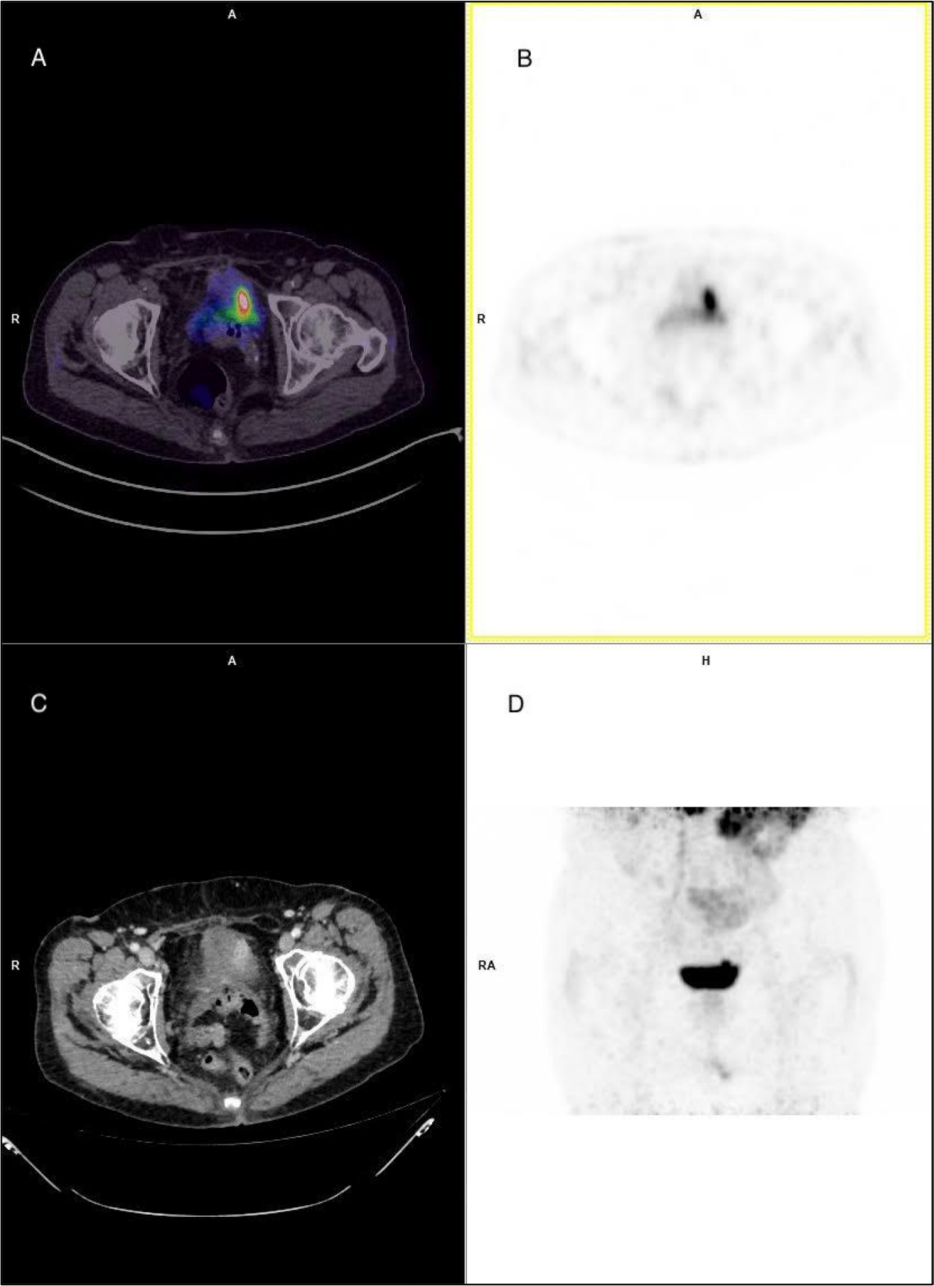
78-year-old patient with high-grade urothelial carcinoma. (A) and (B) Transaxial ^68 ^Ga-PSMA-11 PET/CT fused and ^68 ^Ga-PSMA-11 PET-only images showing focal high PSMA uptake at the site of the primary BCa (left bladder wall); (C) Transaxial contrast-enhanced CT image showing primary BCa enhancing lesion; (D) Maximum Intensity Projection (MIP) image of ^68 ^Ga-PSMA-11 distribution in the pelvic area.
